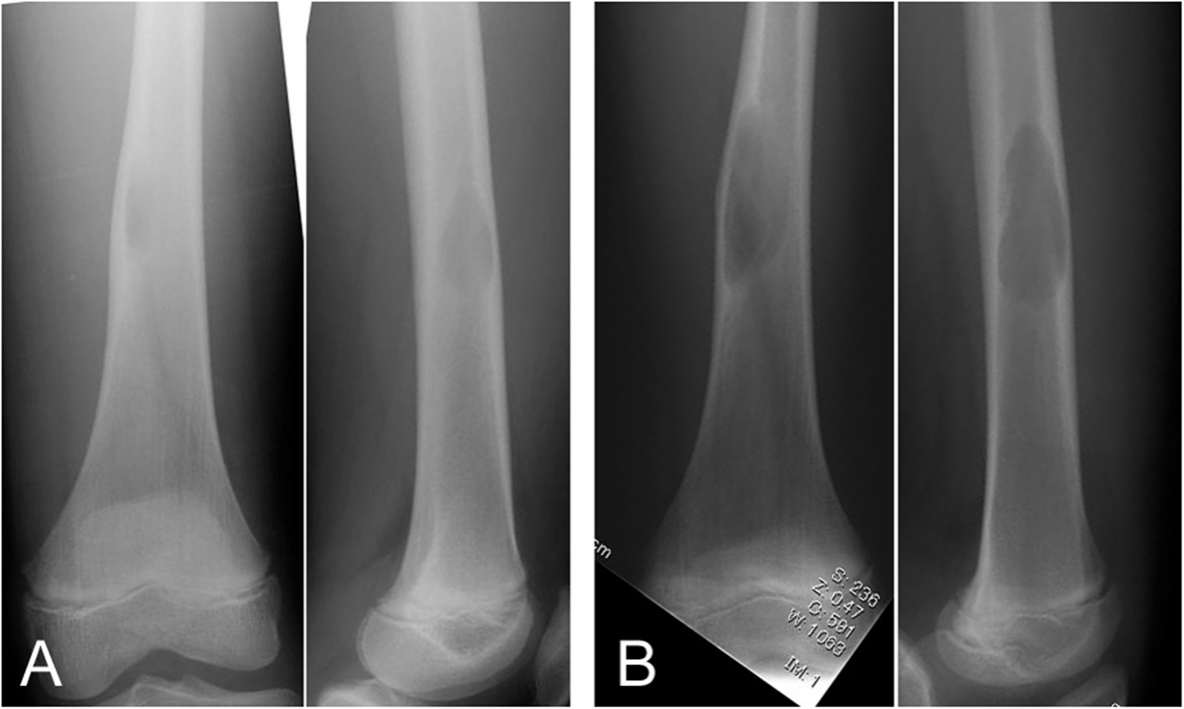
Antero-posterior (left) and lateral (right) plain radiographs show a well-defined osteolytic lesion in the medullary space with cortical thinning (A). The size of the lesion increased over 10 months after the initiation assessment on antero-posterior (left) and lateral (right) views (B).
